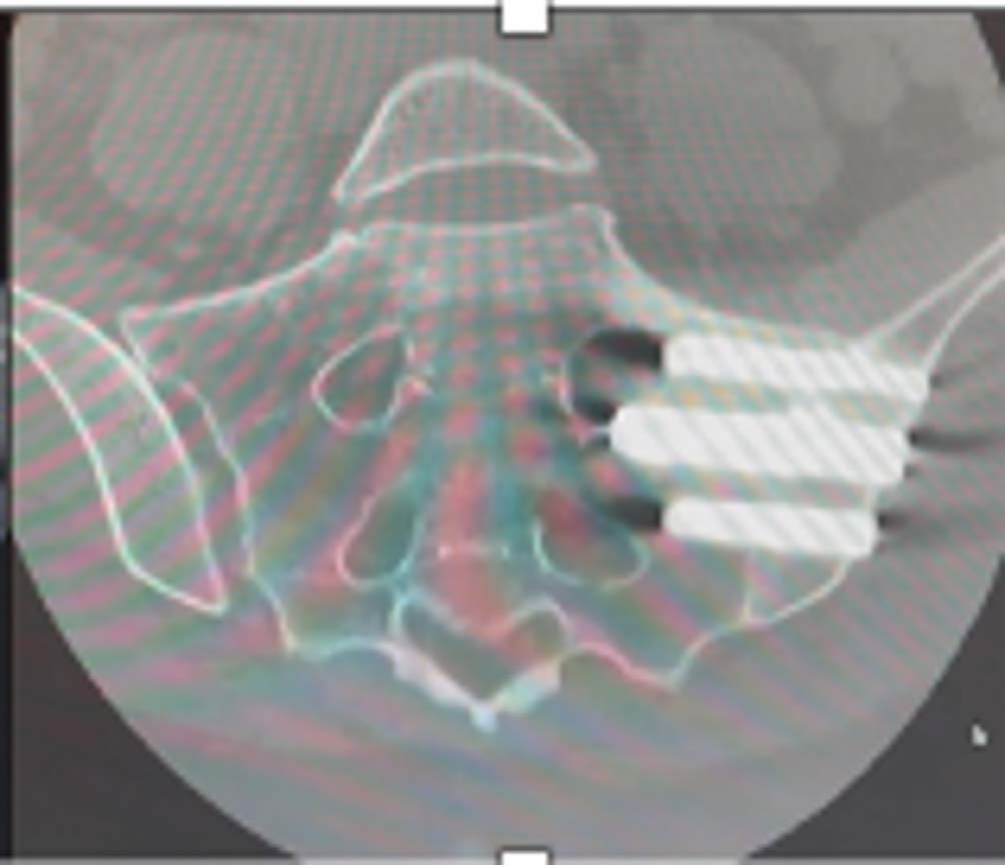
CT scan showing coronal cuts along axis of a dysmorphic sacrum. Note the high angulation of sacral alar and big neural foramina. The implant fixation was limited to Dennis I zone to allow for safe placement of implant.
